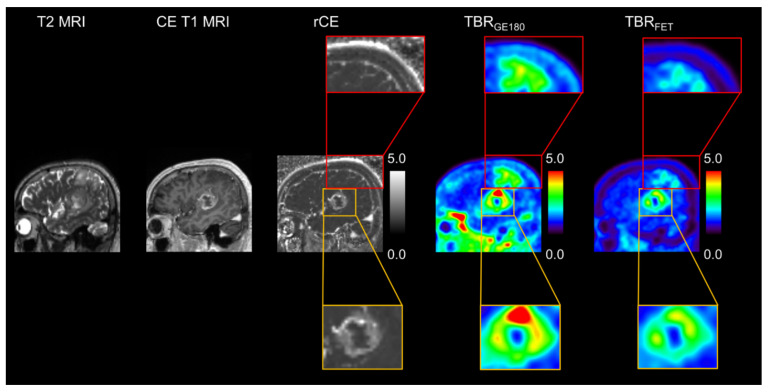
Shown are multi-modal images of one example patient in sagittal view. Depicted are T2-weighted (T2 MRI) and contrast enhanced T1-weighted MRI images (CE T1 MRI) and the generated relative contrast enhancement (rCE) and tumor-to-background ratio (TBR_GE180_, and TBR_FET_) images. Color-bars and intensity ranges are provided for quantitative images. The patient presented with clear tumor specific signal within each modality. In the superior part an additional tumor area without MRI contrast enhancement can be identified using PET, with [^18^F]GE180 PET signal revealing the highest contrast to background.
